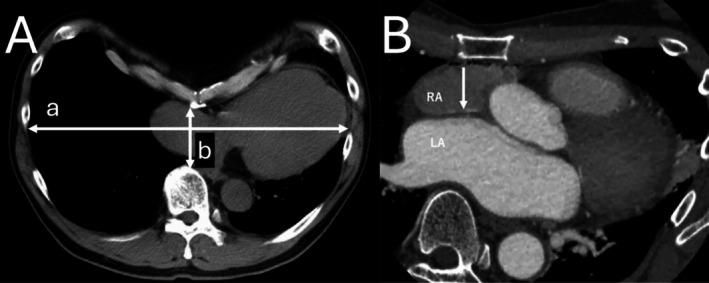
Haller index and Brockenbrough site. (A) Chest computed tomography (CT) suggests severe pectus excavatum. (a) Maximum transverse diameter: 272.84 mm. (b) Minimum anterior–posterior diameter: 51.29 mm. Haller index = 272.84/51.29 = 5.31. Cardiac CT shows a depressed thoracic cage, resulting in significant compression of the left atrium against the vertebral body. Furthermore, the spatial relationship between the inferior vena cava and heart is markedly altered, deviating from the normal anatomical configuration. (B) The white arrow indicates the direction in which the radiofrequency needle was oriented during the Brockenbrough procedure, demonstrating the necessity of advancing the needle toward the vertebral body.
